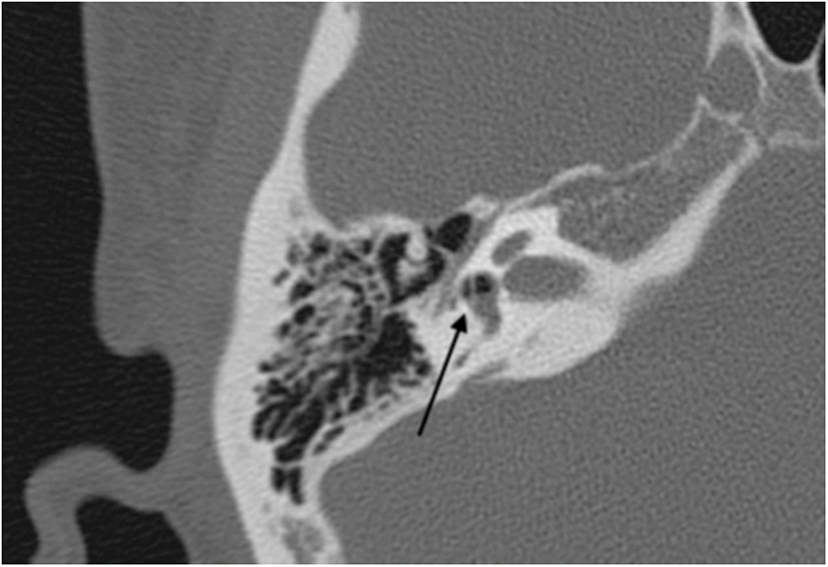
Preoperative CT scan. Air bubbles (arrow) are visible in the vestibule.
